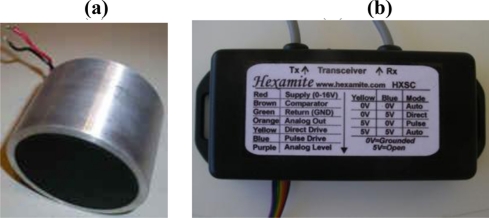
(a) Ultrasonic sensor. (b) Signal conditioner.
